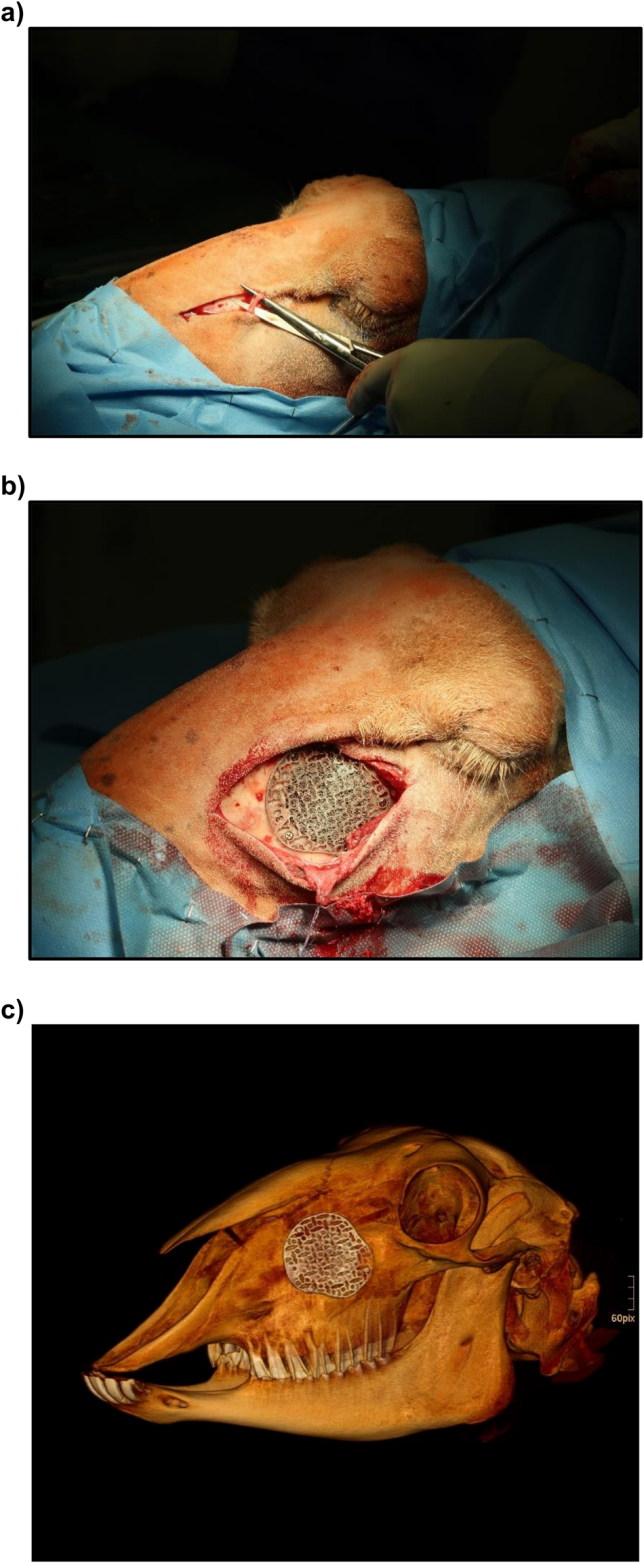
a, b Intraoperative exemplary image after preparation of the angular vein and insertion of an empty scaffold into a 2.4 cm defect in the anterior wall of the maxillary sinus. c Postoperative CT scan showing correct placement of the scaffold
